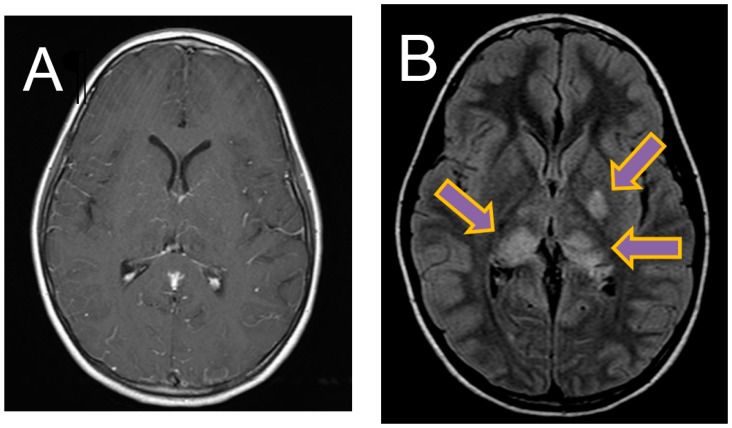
Magnetic resonance imaging (MRI) brain images. (A) Axial T1-weighted image showing diffuse leptomeningeal enhancement. (B) Axial FLAIR image showing FLAIR hyperintensity in left basal ganglia and bilateral thalami.
